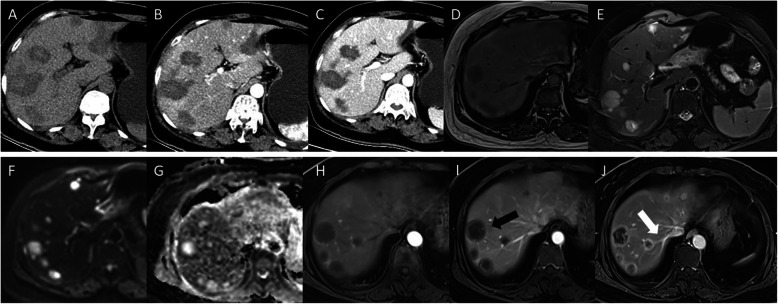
A 52-year-old woman with EHE. The nonenhanced CT scan (a) reveals multiple ill-defined homogeneous hypoattenuated lesions. The lesions demonstrate marginal enhancement in the arterial phase (b) and heterogeneous progressive centripetal enhancement in the portal vein (c). The MRI scan (d, T1WI in-phase; e, fat-suppressed T2WI; f, DWI; g, ADC map) shows hypointensity on T1WI, hyperintensity on T2WI and high signal intensity on DWI and the ADC map. The enhancement pattern on dynamic contrast-enhanced MRI (h, arterial phase; i, portal venous phase; j, delayed phase) corresponds with that on CT. The lesion presents with the halo sign (black arrow) and lollipop sign (white arrow)
